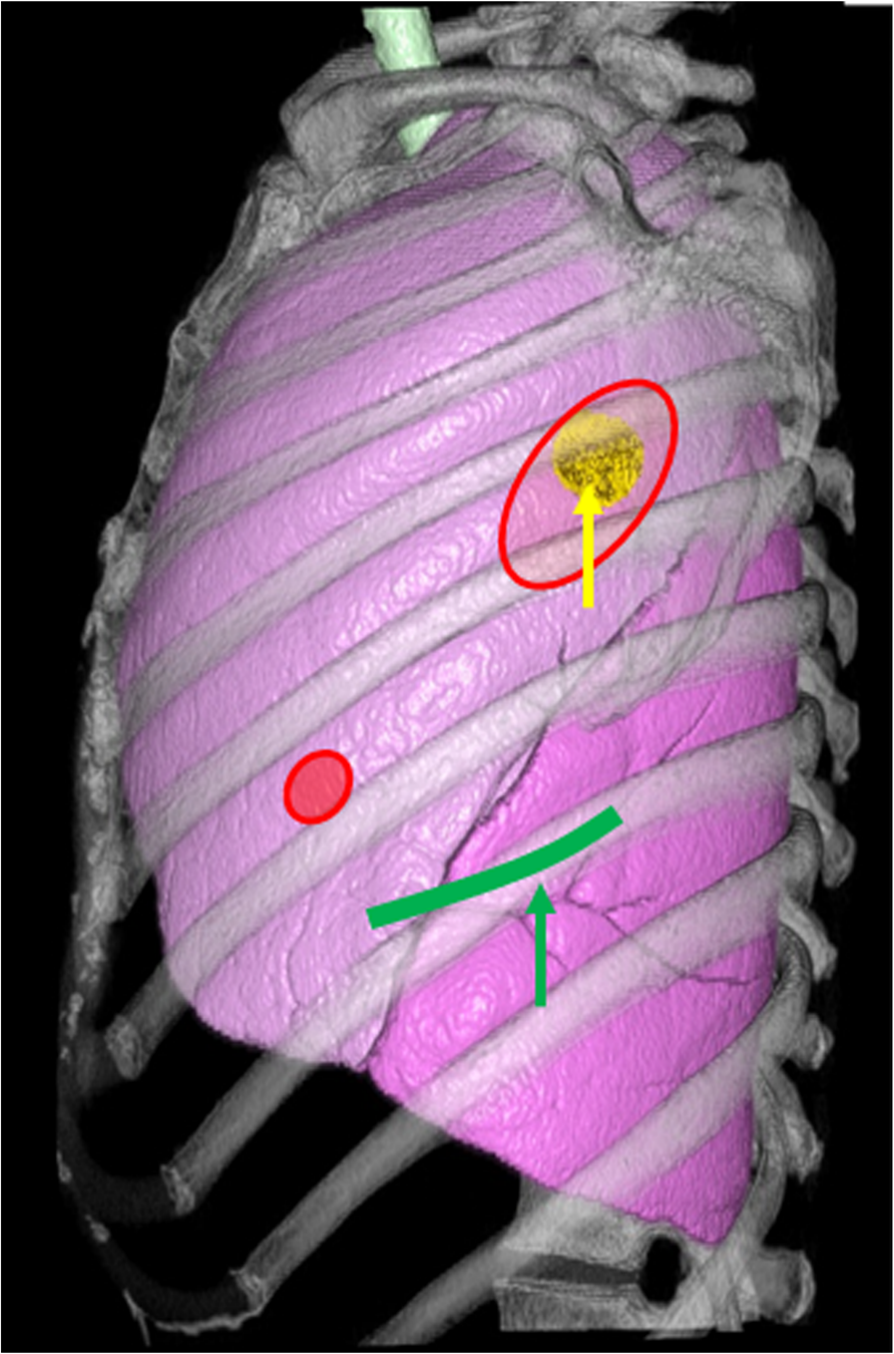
Three-dimensional image reconstructed from computed tomography with operative images. Thoracoscopic excision of the mass was done with two ports (*small red circle*, 3 mm port; *red large circle*, 2 cm access port). The cyst (*yellow arrow*) was far from the previous operative scar (*green curved line*). The lower lobe of the left lung had a fold (*green arrow*) that had been made from past wedge resection
